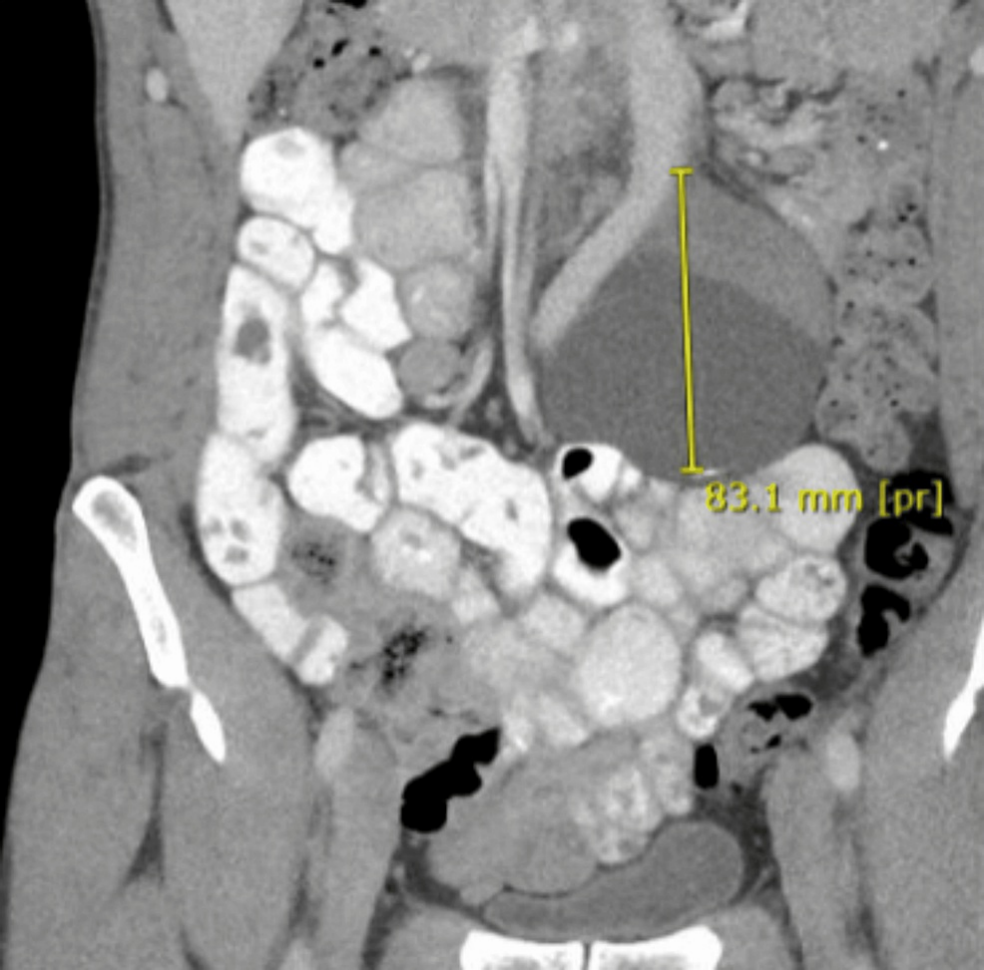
CT scan: coronal view of the para-aortic mass demonstrating its proximity to the aortic bifurcation and its heterogeneous components
